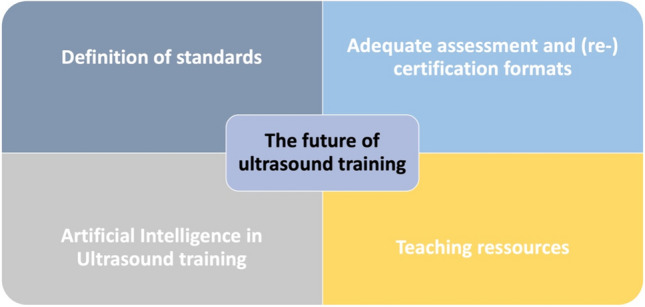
Main focus of innovative approaches for future ultrasound
